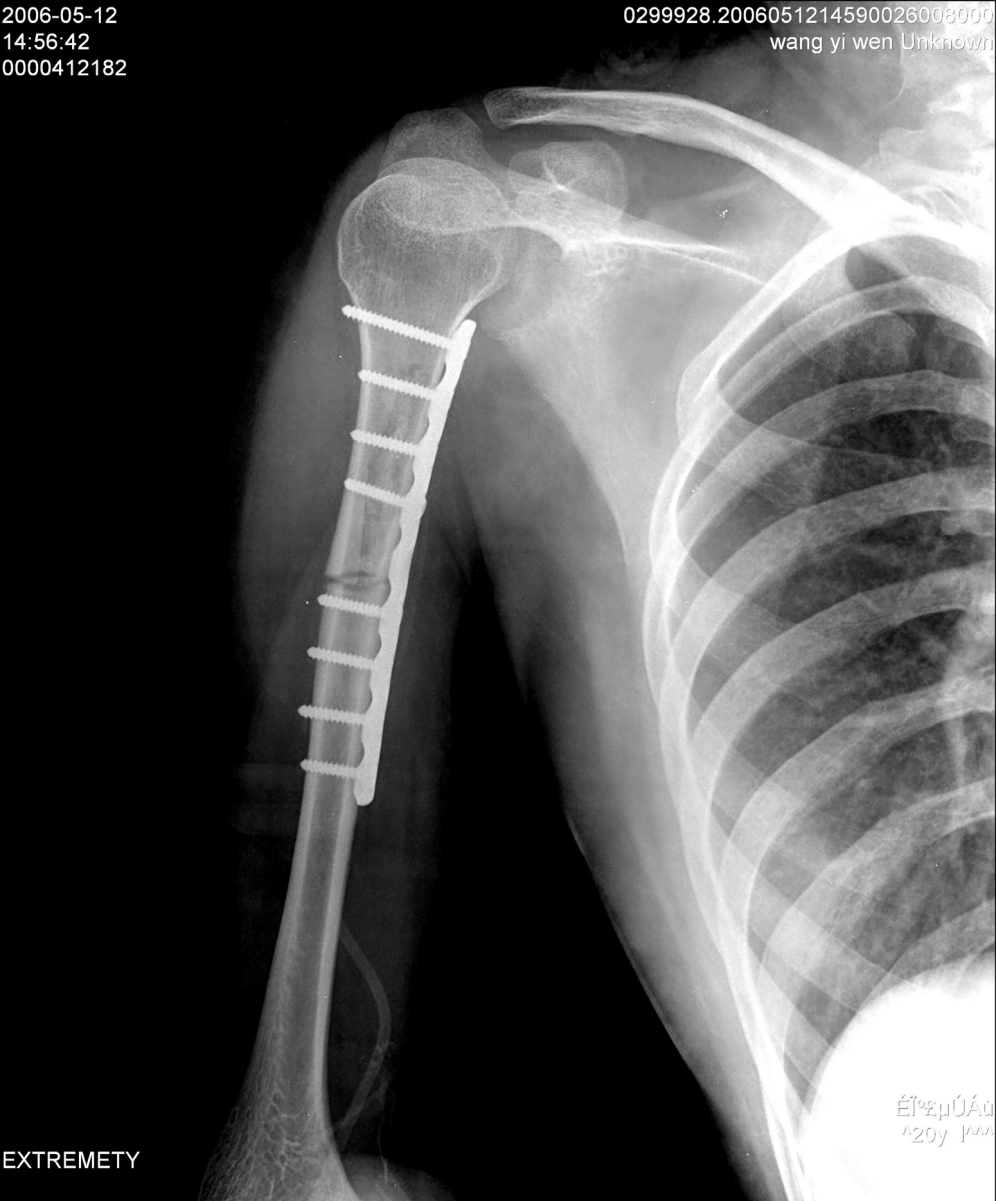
Radiograph after operation.
